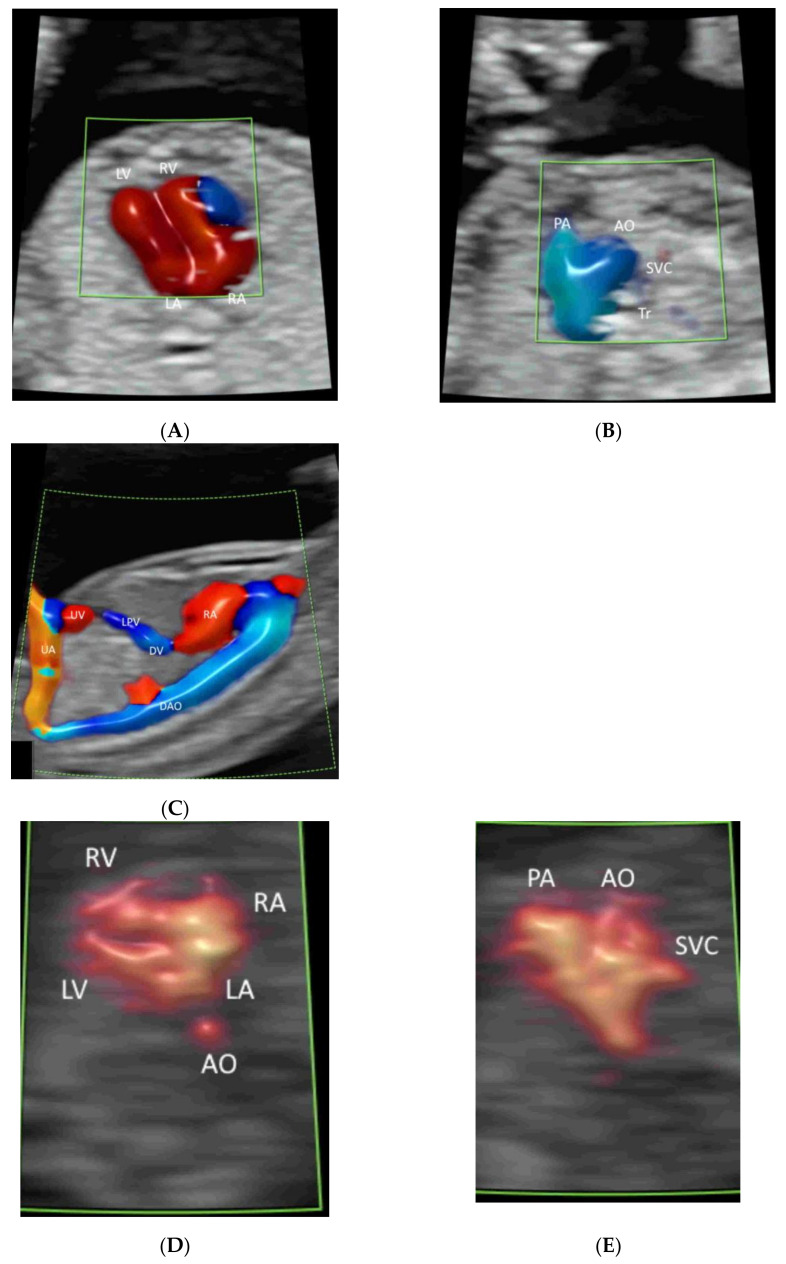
Color Doppler flow imaging with radiant flow showing the four-chamber view of the normal fetal heart (A), three-vessel trachea view (B), and ductus venosus (DV) connecting to the heart (C), at 12–13 weeks. Microvascular flow imaging of a normal fetal heart at 11 weeks’ gestation showing the four-chamber view (D) and three-vessel view (E). RA, right atrium; RV, right ventricle; LA, left atrium; LV, left ventricle; PA, pulmonary artery; AO, aorta; SVC, superior vena cava; Tr, trachea; LPV, left portal vein; UA, umbilical artery; UV, umbilical vein; DAO, descending aorta.
